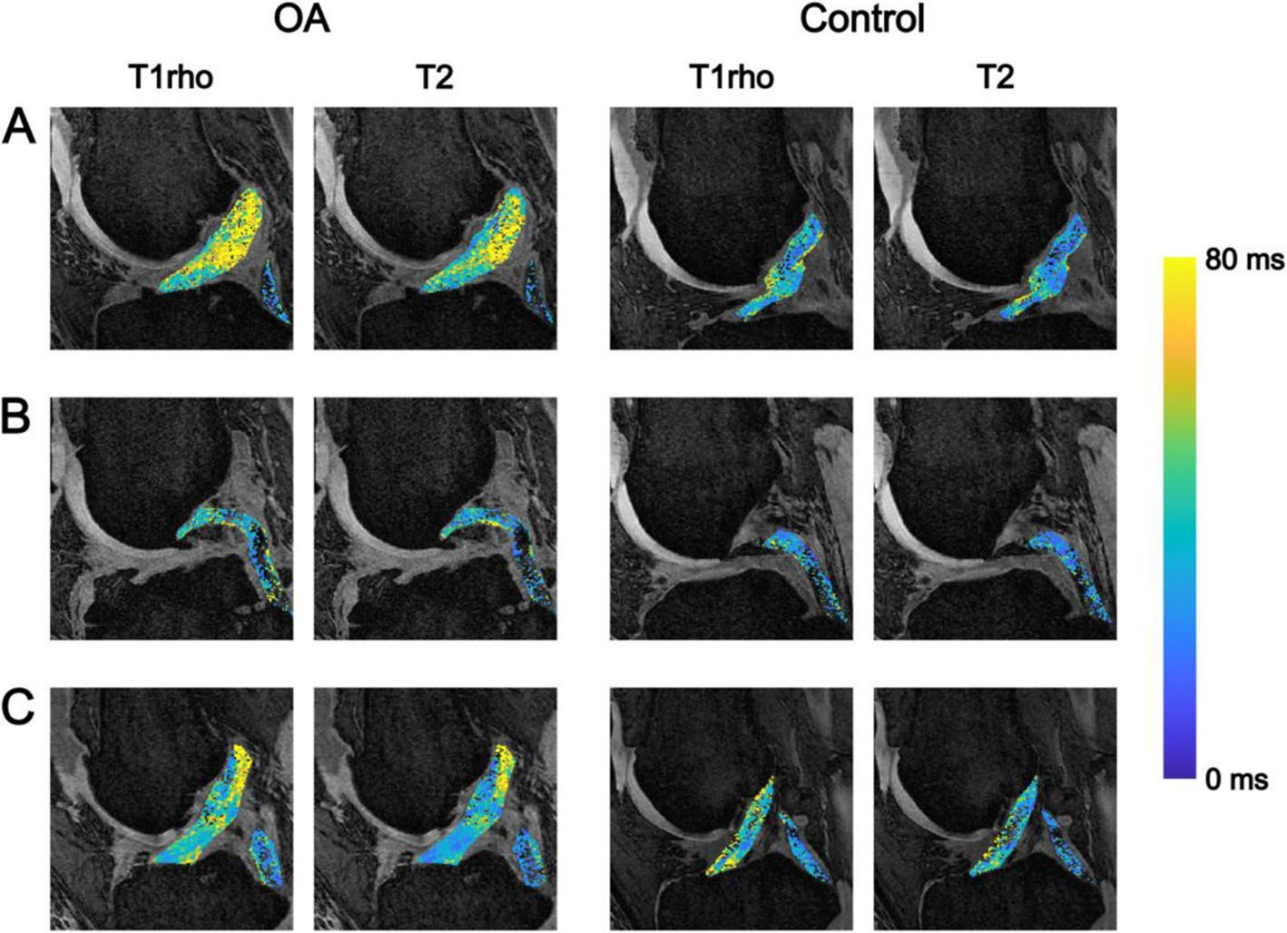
A Representative sagittal 3D spoiled gradient echo MRI images of the ACL of an OA (left) and healthy (right) subject with overlaid T1rho colormaps. Note higher and more heterogeneous values of T1rho in the OA subject. B As above but with T1rho colormaps of the PCL. Note higher T1rho values in proximal part of the PCL in the OA subject. C Variation in T1rho within the cruciate ligaments of an OA (left) and healthy (right) subject. Note increased variation/heterogeneity of T1rho values throughout the ACL of the OA subject
